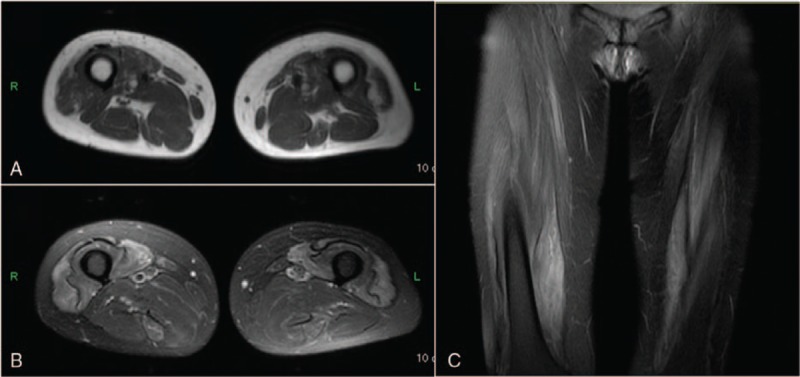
Magnetic resonance imaging of bilateral femoral quadriceps revealed normal intensity on T1-weighted image (A) and high intensity on T2-weighted image (B) and fat-suppressed T2-weighted image (C).
